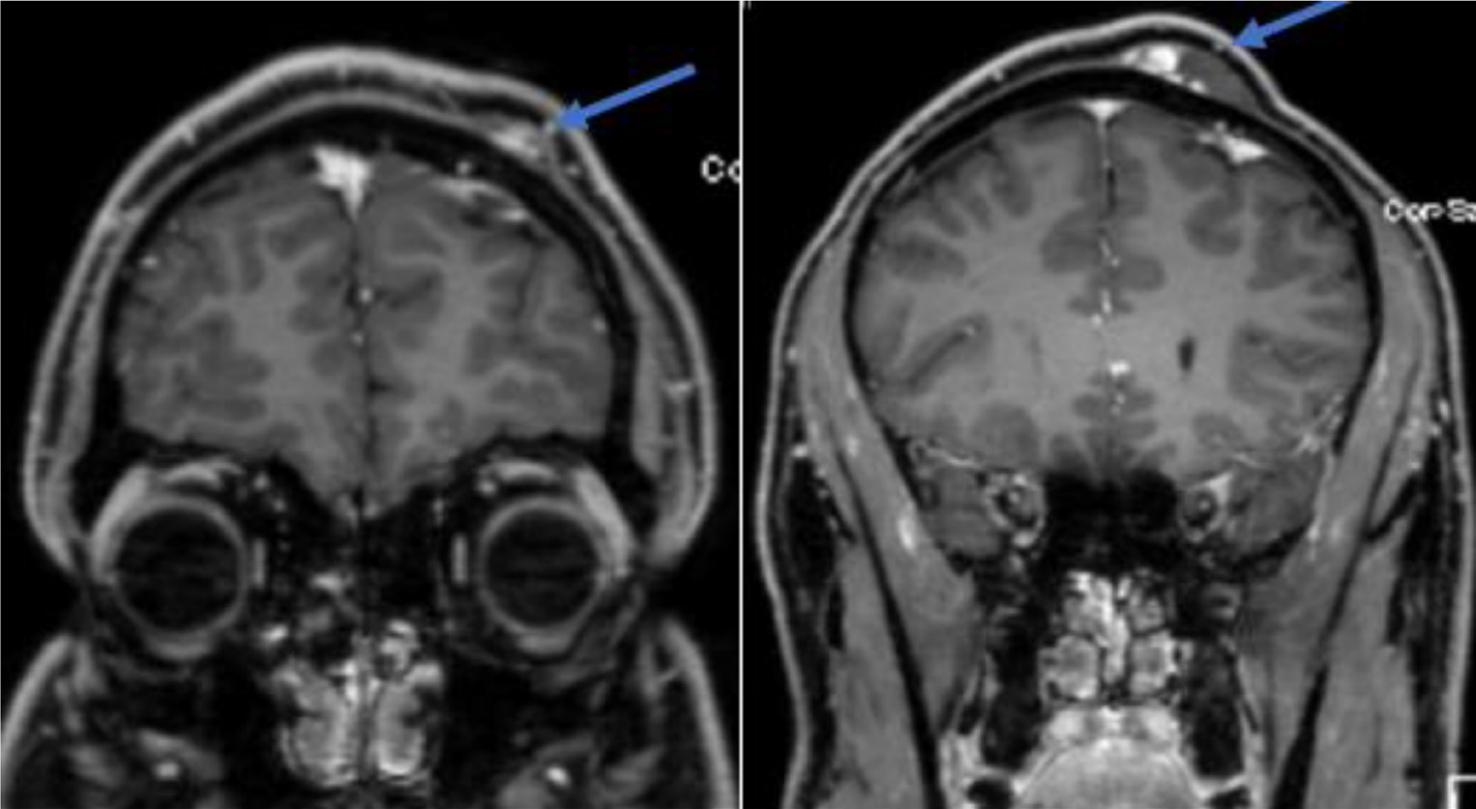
MRI T1 with contrast showing inhomogeneous contrast enhancement of the mass (blow arrows).
